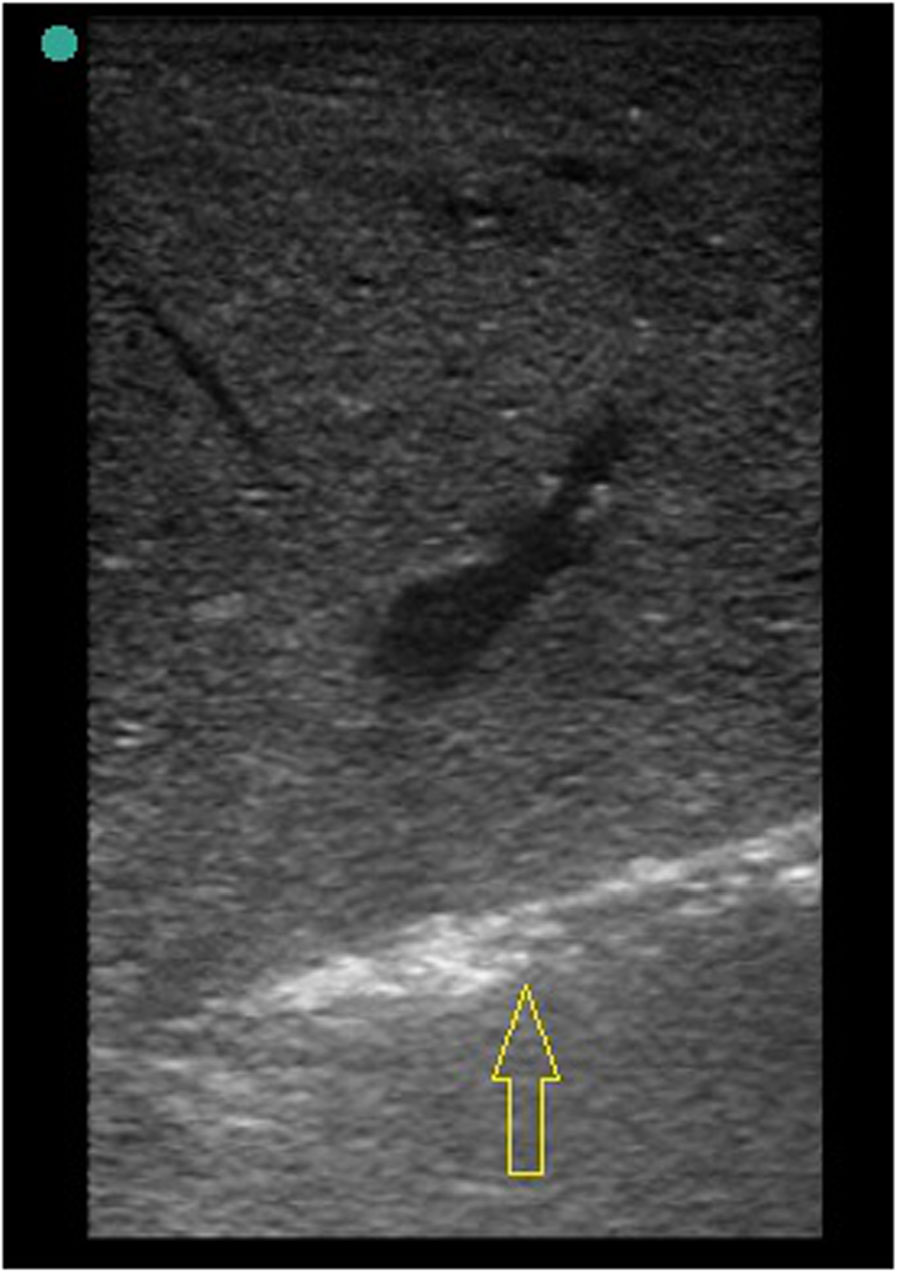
Sonographic image of flooded lung after biopsy shutter release so that tissue is sampled out of the focal zone (*arrow*)
